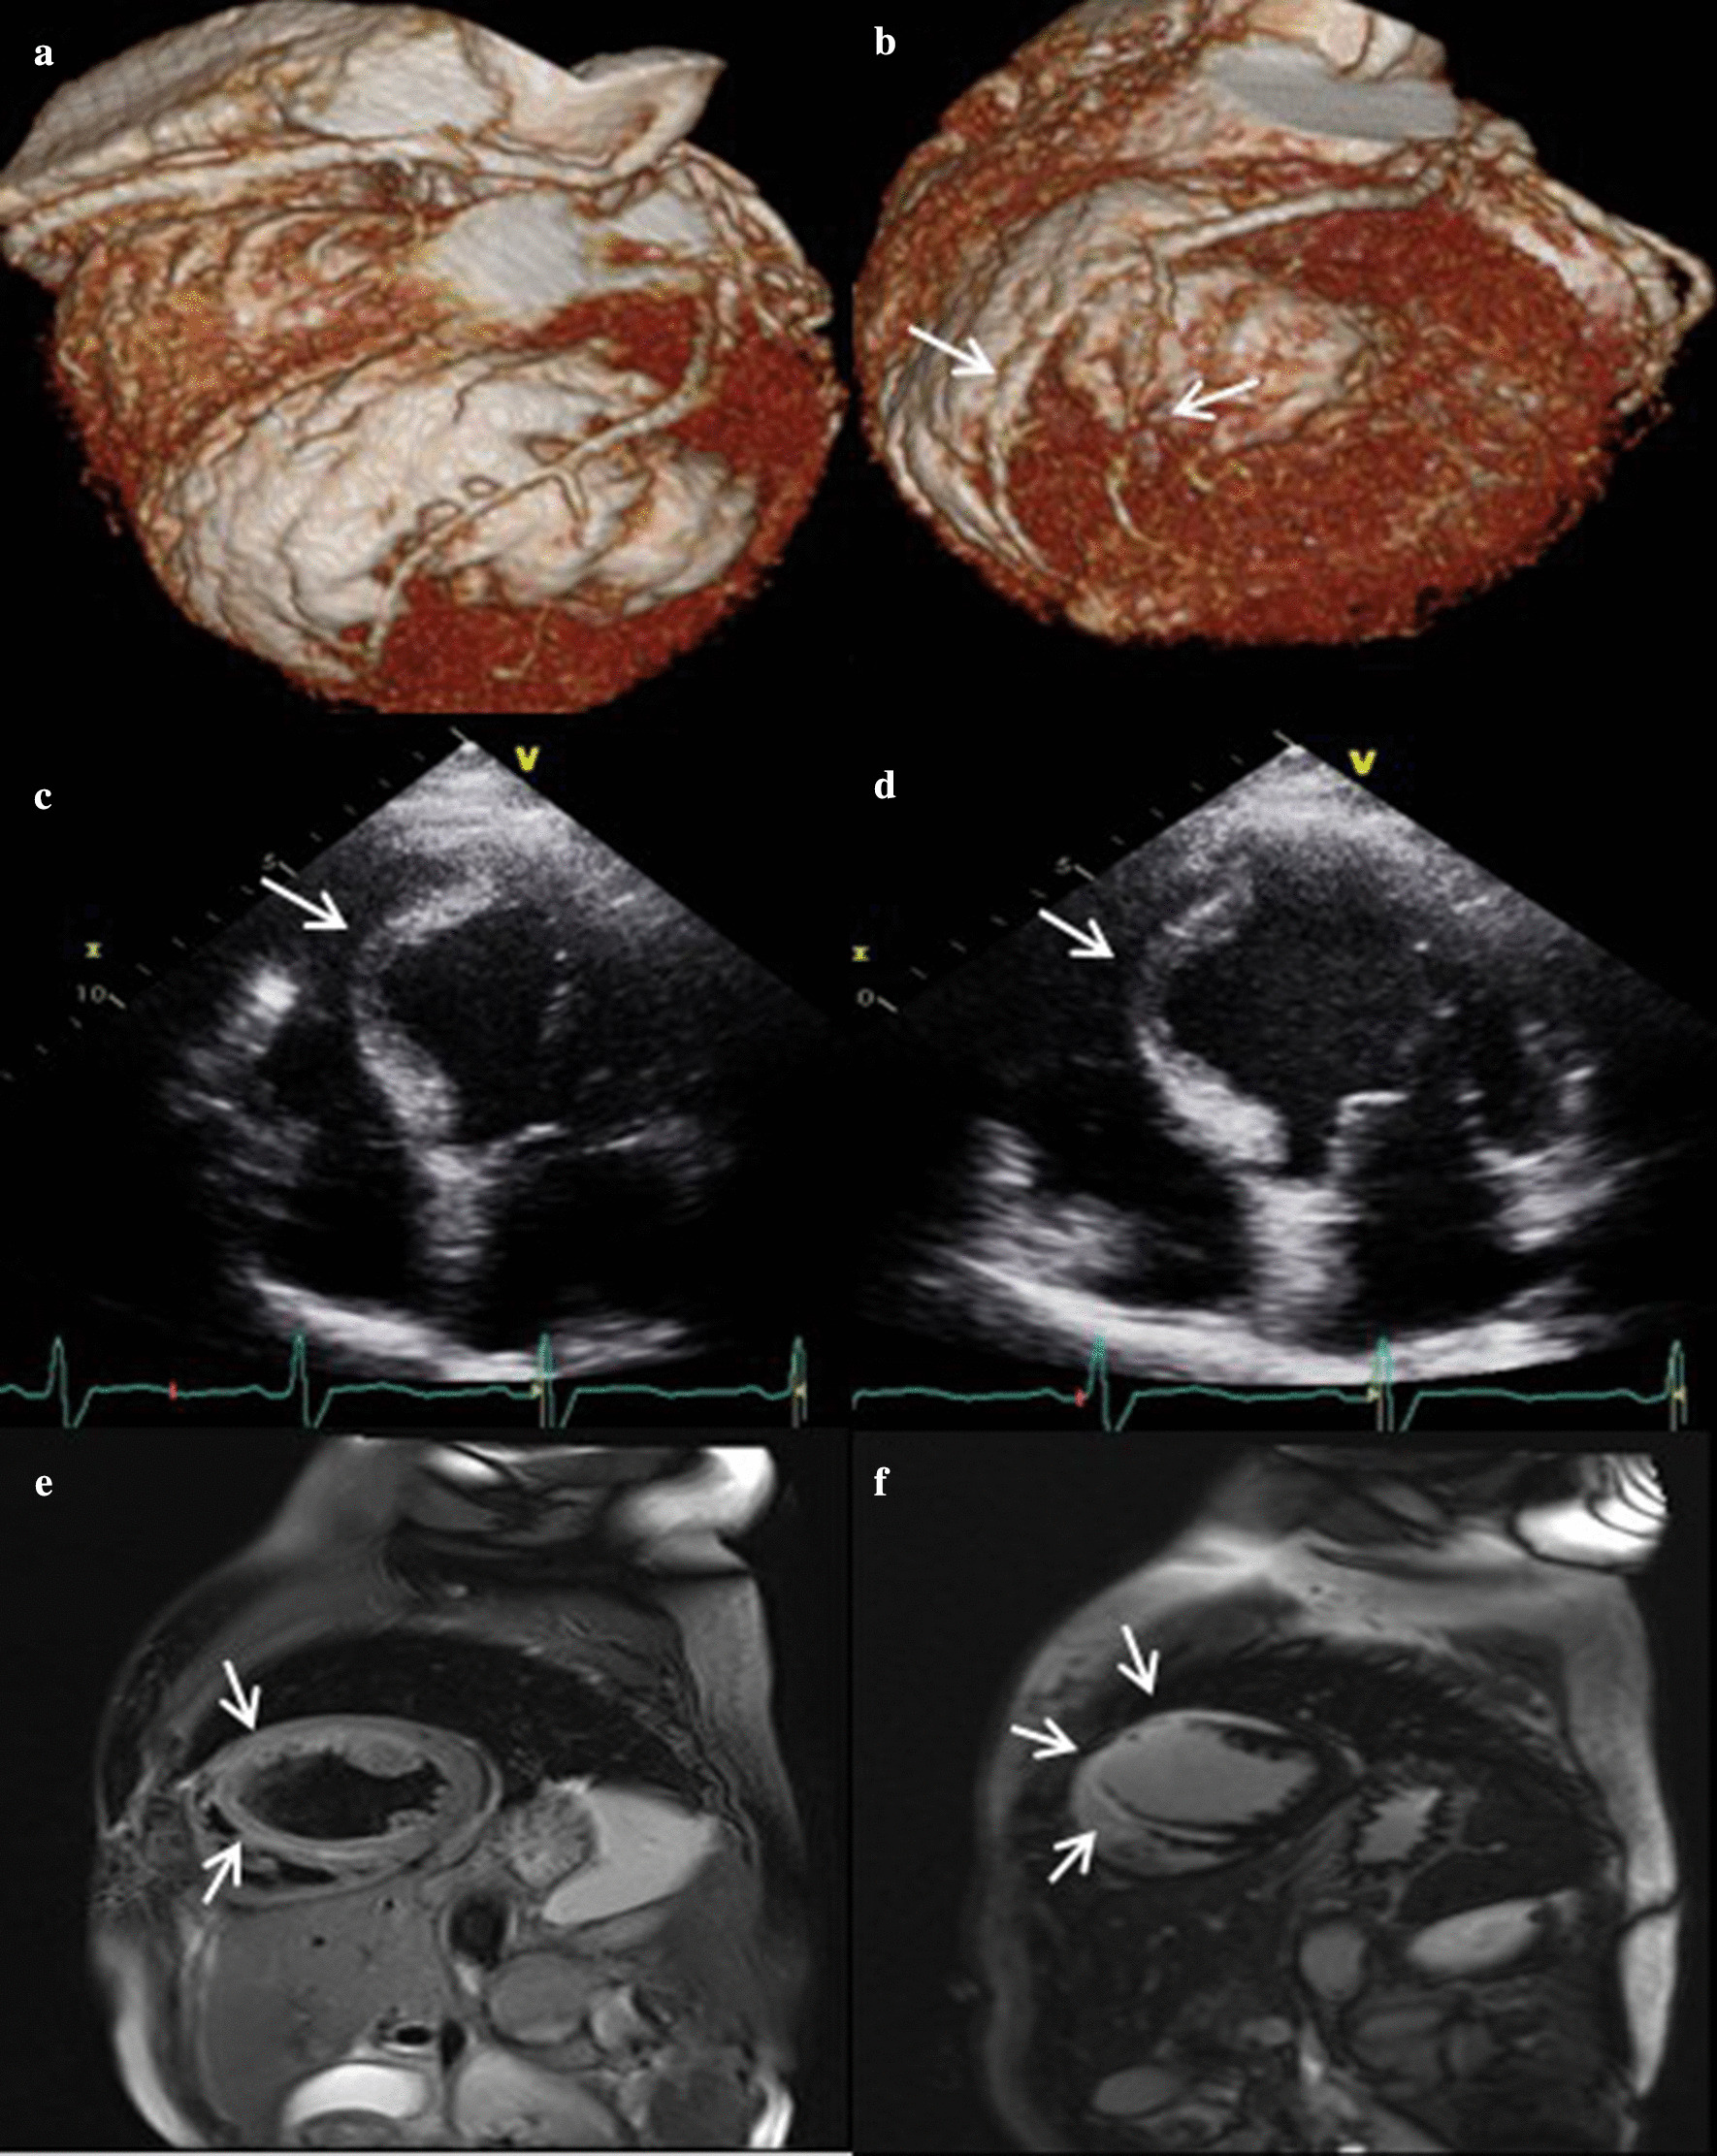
3D volume rendered reconstruction of the heart and coronary arteries showed high take off of the right coronary artery (a) and distal segment of the left anterior descending coronary artery and diagonal branch with a superficial intra-myocardial course (arrow) (b). Resting echocardiography showed focal septal wall motion abnormalities called septal buckling (arrow) at end systole (c) and early diastole (d). T2-weighted heart MRI image showed myocardial wall thinning and subendocardial myocardial oedema (arrow) in the mid-ventricular to apical anterior and septal regions (e). Late gadolinium enhancement imaging showed a high signal intensity in the same region (f)
